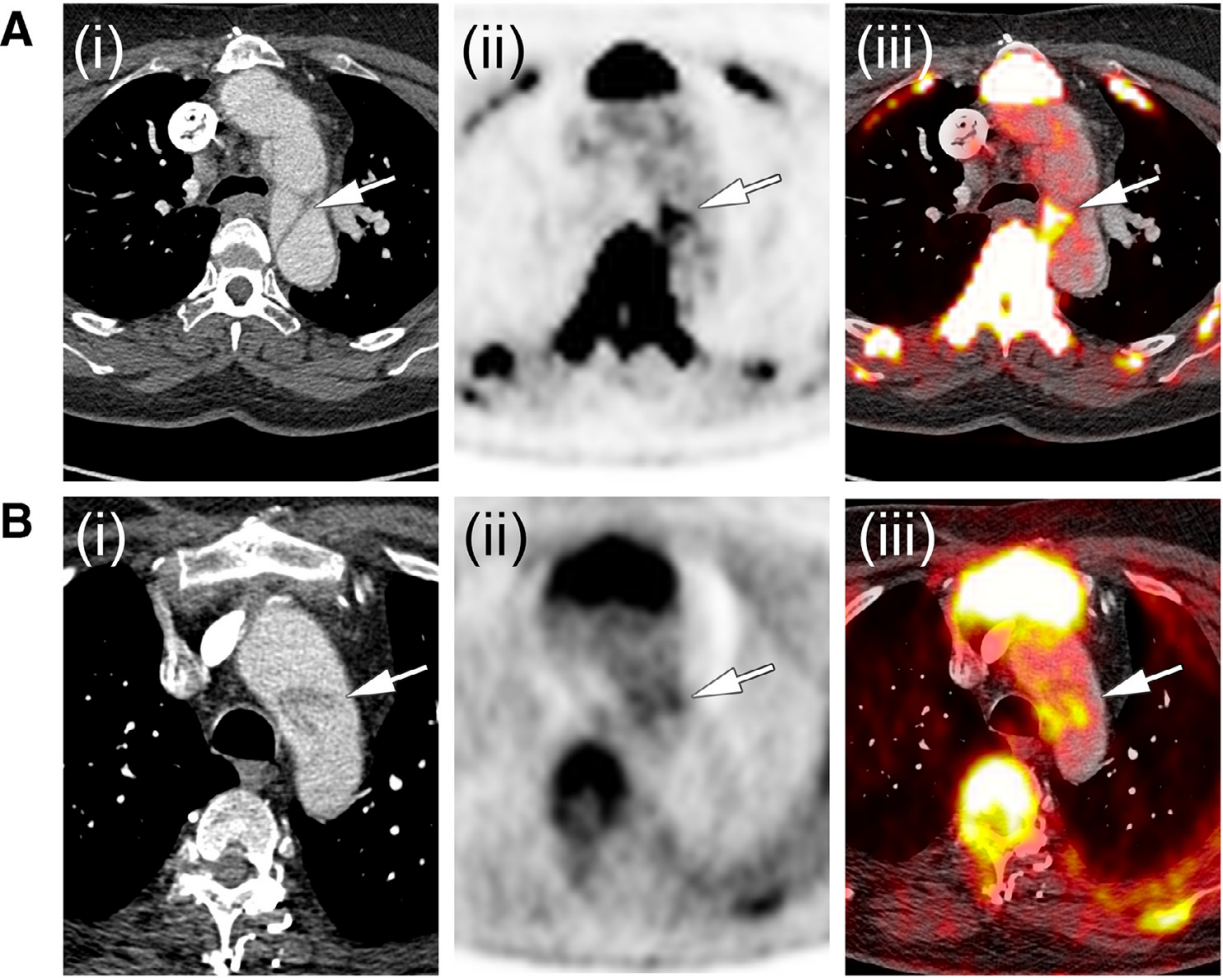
^18^F-Sodium Fluoride following aortic dissection. (i) Contrast enhanced computed tomography, (ii) ^18^F-Sodium Fluoride positron emission tomography and (iii) combined ^18^F-Sodium Fluoride positron emission tomography/ computed tomography in a patient with (A) hypertension-induced aortic dissection and (B) in a patient with Marfan Syndrome.
